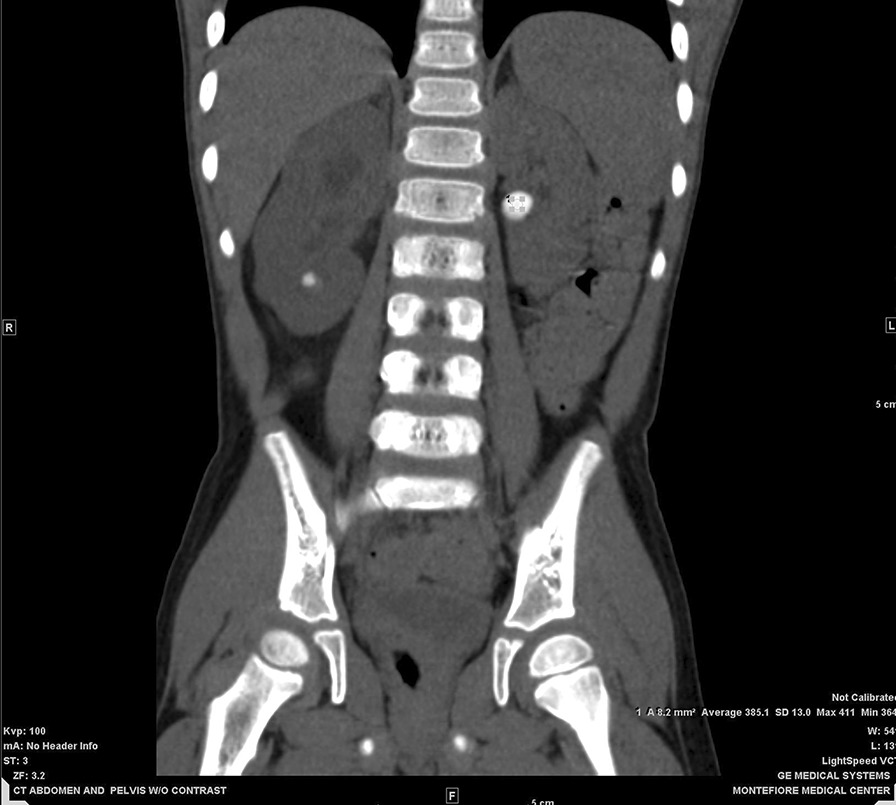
In vivo CT of xanthine urinary stones: coronal CT image showing a stone within the left kidney along with measurement of CT number utilizing region of interest sampling
